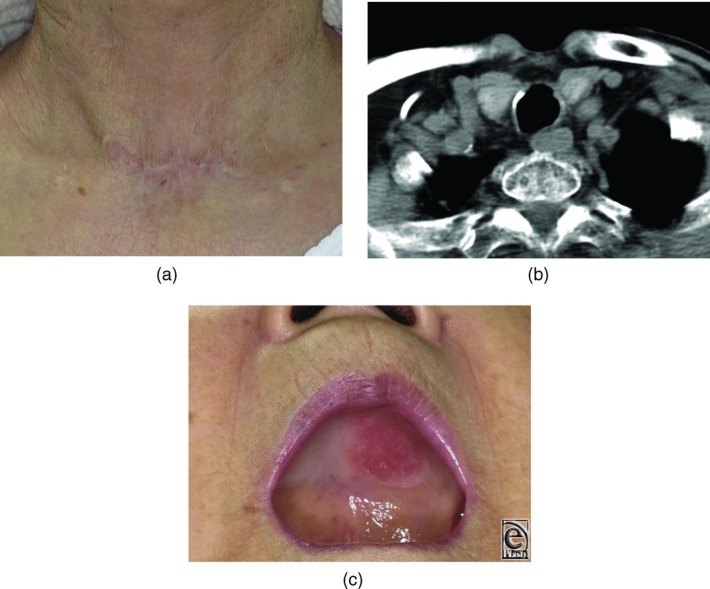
Findings 1 year after surgery. (a) The neck scar is inconspicuous. (b) Computed tomographic scan showing complete closure of the tracheocutaneous fistula without stenosis. (c) Skin graft on the palate is durable, and a full denture could be worn as before.
